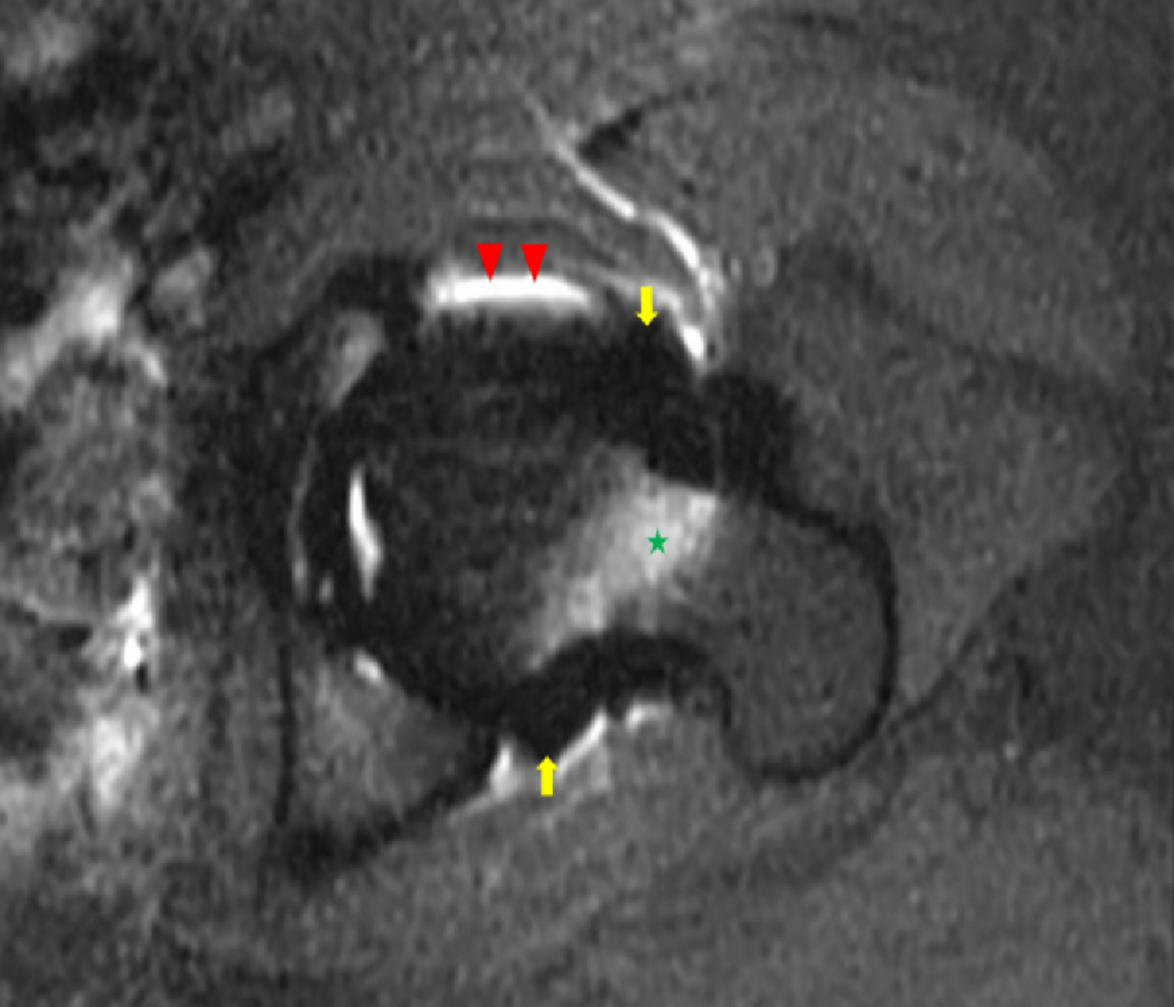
Axial T1 weighted fat-saturated magnetic resonance image of the left hip A T1 weighted axial fat-saturated magnetic resonance image of the left hip exhibits a signal void surrounding the left hip (yellow arrows), conforming to the shape of the joint capsule, status post-injection for magnetic resonance arthrography. There is an incidental note of altered fat saturation at the anterior and posterior joint capsule margins (red arrowheads) and within the marrow of the femoral neck (green star).
